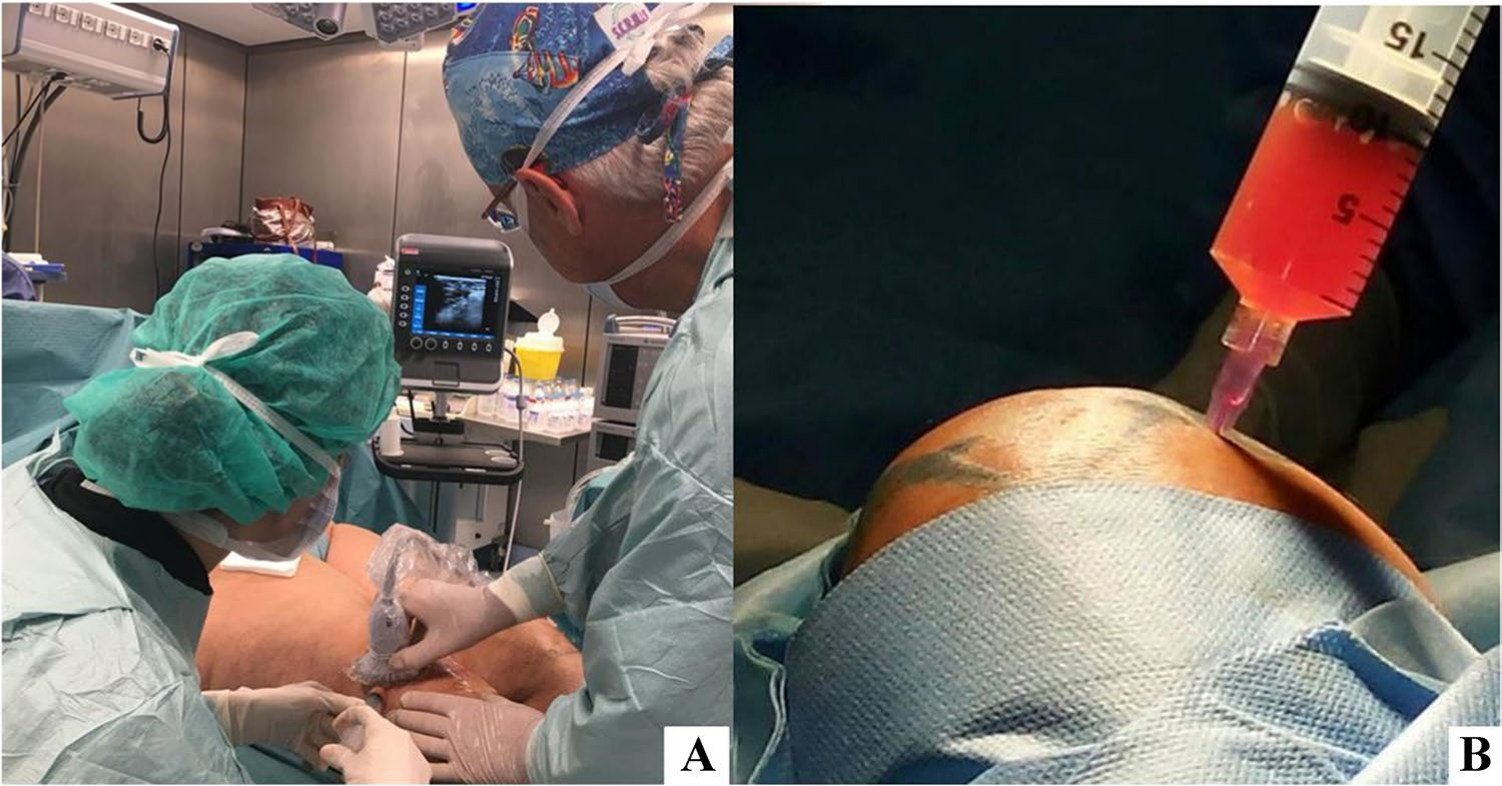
Knee joint infiltration A ultrasound guided injection; B detail of intra-articular injection (courtesy of T. Nguyen)
